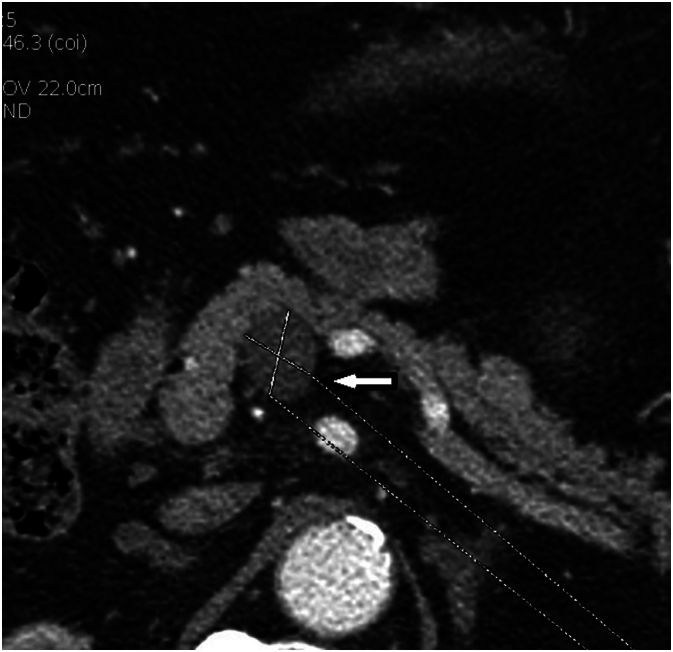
Post-contrast abdominal CT scan showing a well-defined hypodense lesion measuring 20 × 21 mm in the pancreatic body. The lesion is clearly delineated from the surrounding pancreatic parenchyma.
